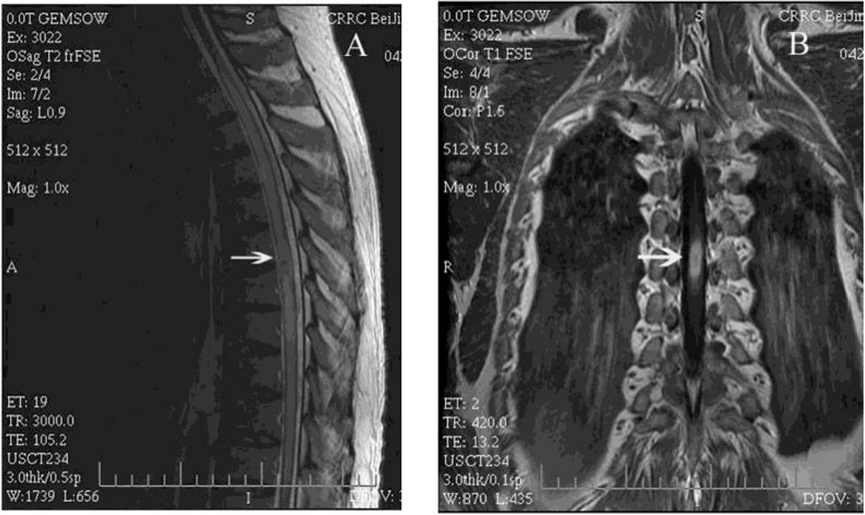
The MRI images of the thoracic spine at the time of neurologic deterioration. A) Sagittal T_2_-weighted MRI image shows an increase in the size of the lesion. B) Coronal T_1_-weighted MRI image shows an enlarged ring-like enhancing intramedullary lesion (40 × 10 × 7 mm) with gadolinium.
